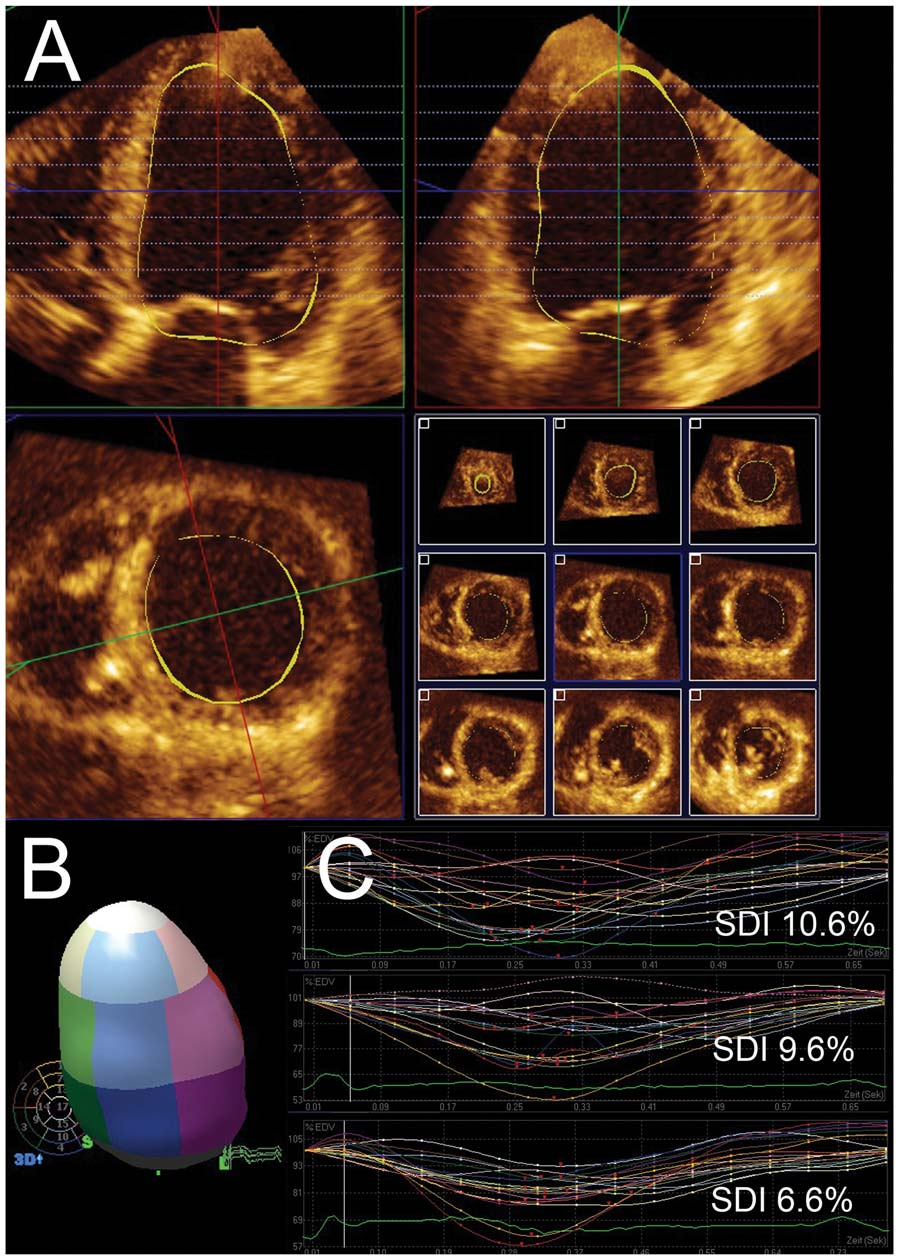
3D-echocardiography guided optimization of cardiac resynchronization therapy. Patient with ischemic cardiomyopathy, severly reduced systolic left ventricular (LV)-function (EF 16.8%), left bundle branch block and dyspnea on exertion (NYHA class III) selected for cardiac resynchronisation therapy (CRT) before biventricular pacemaker implantation: A) tracing of the LV endocardial boundary in the multiple apical and short axis views. From these datasets a volumetric model of the left ventricle is drawn (B), and time-volume curves are automatically integrated for each segment: C) time-volume curves of the patient before pacemaker implantation (upper panel); immediately after implantation (middle panel); after complete echocardiographic optimization (lower panel). Shown are the respective SDI values after each optimization step.
